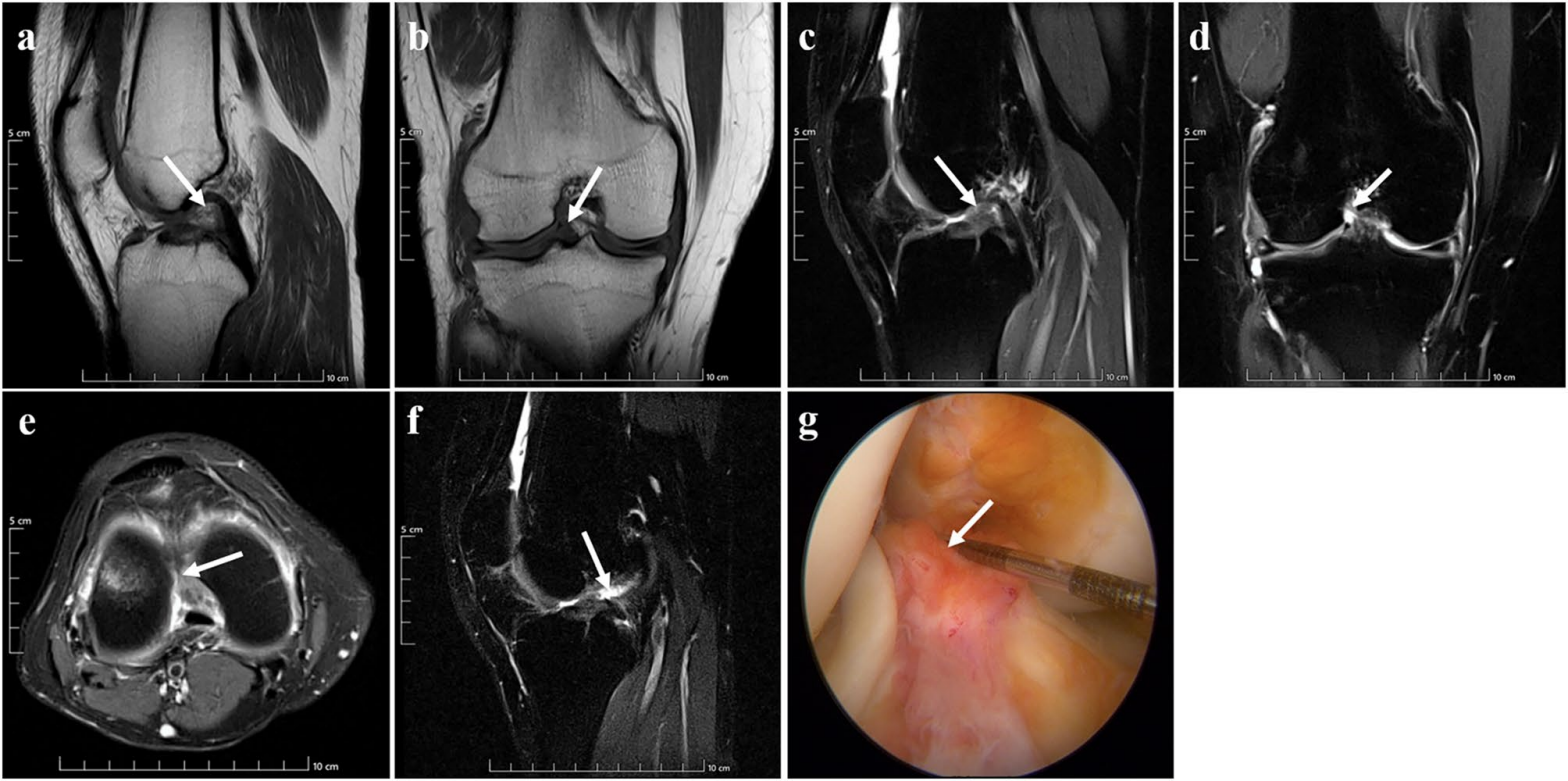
Grade III injury presence on MRI and arthroscopy of a 23-years-old woman. The absence of ligament integrity and continuity were shown on sagittal, and coronal T_1_WI (arrows on (a and b)). Remaining filamentous ACL with surrounding liquid (arrows) still could be seen on sagittal PDWI (c), coronal PDWI (d), and axial PDWI (e), which would cause misdiagnosis. The ligament was completely separated into two parts and the two damaged ends with their intermediate filled fluids (arrow) were displayed on the new modified DPP-TSO-Sag-FS-PDWI (f). Arthroscopy found the ligament was divided into two parts without continuity and completely separated, one of the damaged edges faced up (arrow on (g)).
